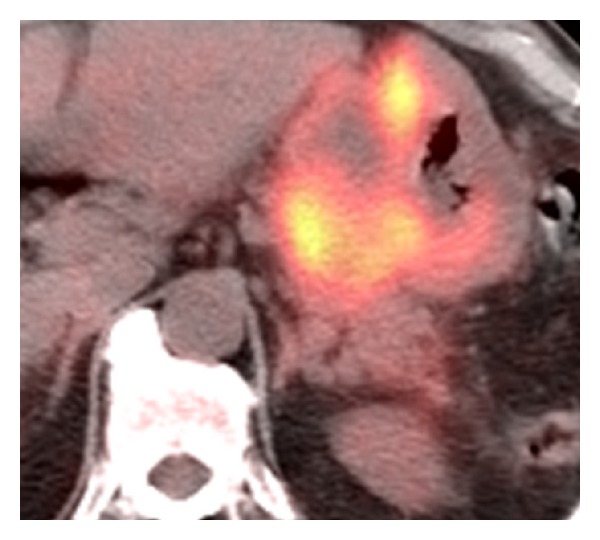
Staging positron emission tomography/computer tomography (PET/CT) of the chest, abdomen, and pelvis revealed fludeoxyglucose (FDG) uptake along the gastric lumen with maximum standardized uptake value (SUV) of 6.5 and increased uptake along the mesentery and cecum with maximum SUV of 5.6 and 5.8, respectively.
